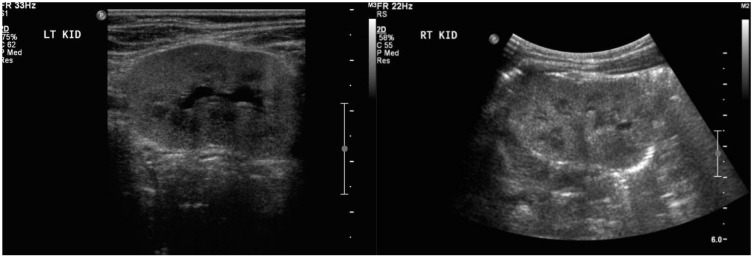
Ultrasound images of baby’s left and right kidney at 6 weeks of age.
